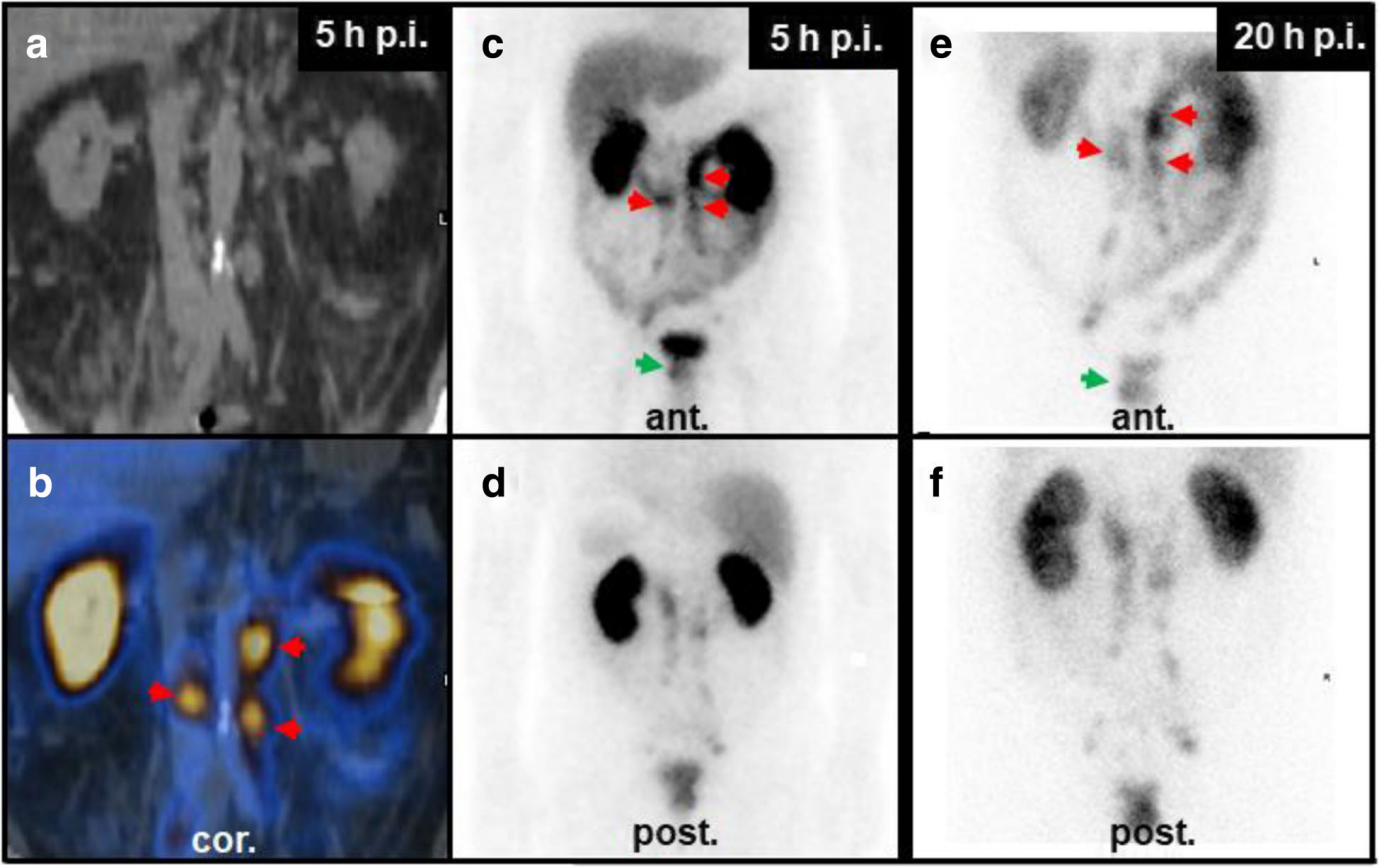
SPECT/CT at 5 h post injection (p.i.) and pairs of ventral/dorsal planar images at 5 h and 20 h p.i. of [^99m^Tc]Tc-PSMA-I&S. a, b SPECT/CT suggested paraaortic lymph node metastases, but the planar scintigraphy (c, d) shows significant hepatobillary and gastrointestinal uptake and suggests urinary secretion. To further distinguish between tumor uptake in paraaortic lymph nodes and unspecific uptake in the ureter (red arrows), a late planar image was taken. e, f Planar scintigraphy at 20 h p.i. Paraaortic uptake remained high and was thus classified as lymph node metastases (red arrows). Note: there was a tracer accumulation in the primary tumor over time, resulting in a better contrast between unspecific bladder signal and the [^99m^Tc]Tc-PSMA-I&S uptake in the prostate (green arrows). Late imaging was restricted to a single bed planar scintigraphy because the low activity at 20 h p.i. would have led to inappropriately high examination times (~ 60 min for planar whole body scintigraphy and whole body SPECT/CT)
